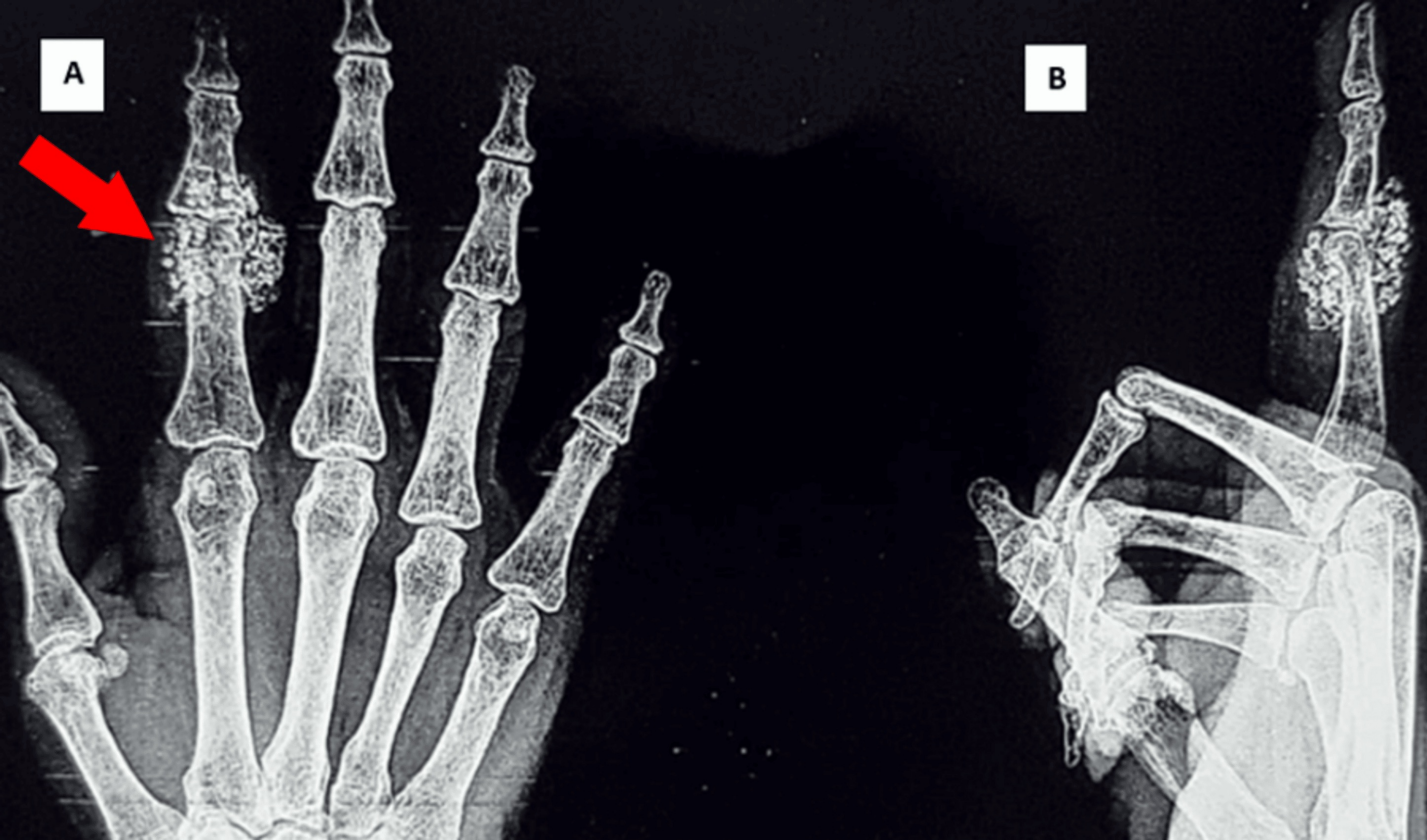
X-ray AP/laterial views of the right hand 1A: X-ray AP view of the right hand with a red arrow showing calcifications around the proximal interphalangeal joint of the index finger. 1B: X-ray lateral view of the right index finger showing calcifications.
